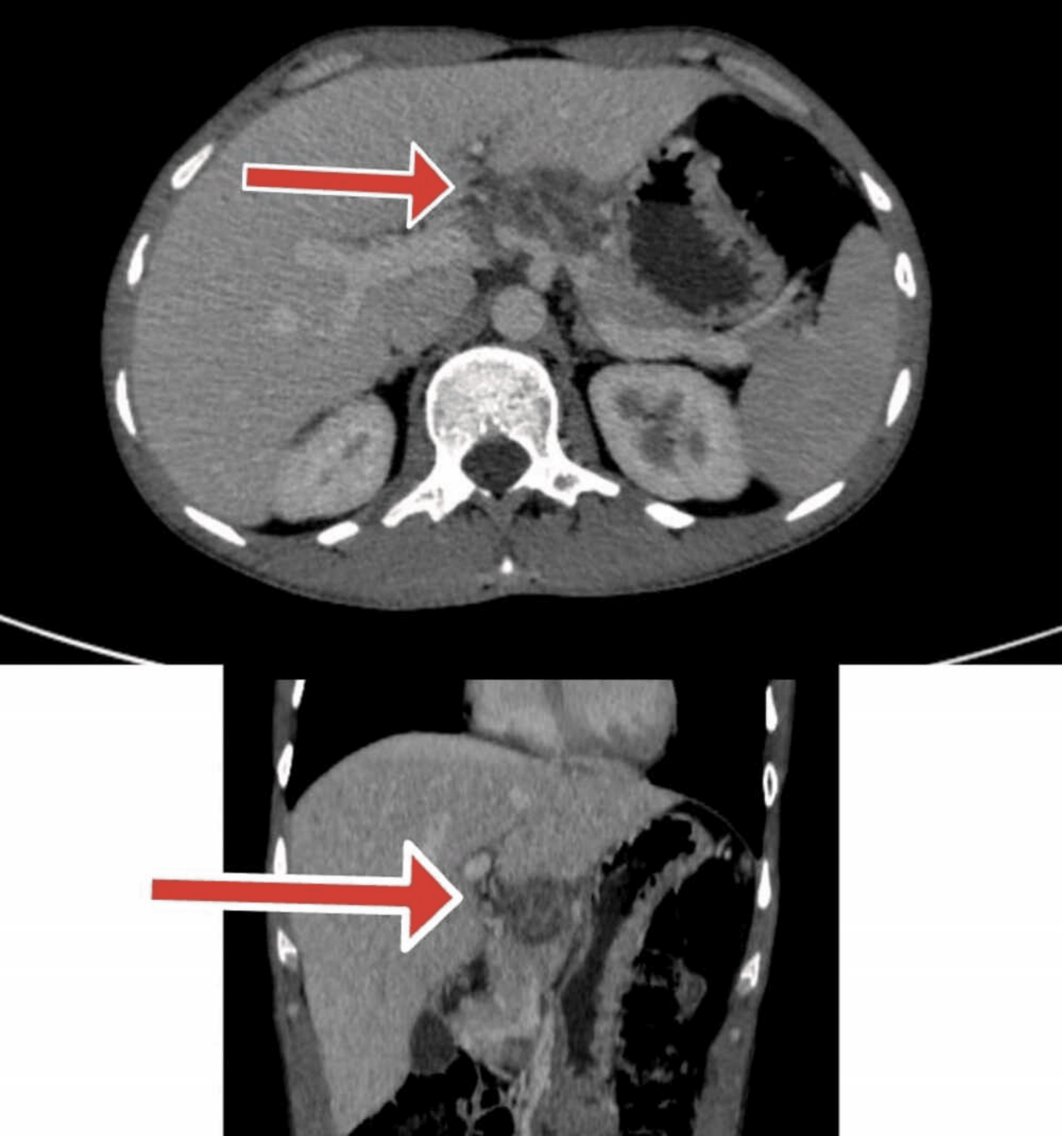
CT abdominal scan showing the hypoechoic lesion indicative of pancreatic mass Red arrows indicate the pancreatic mass. CT: computed tomography
